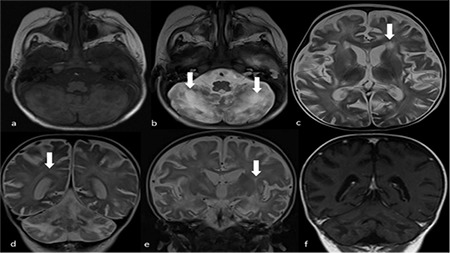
Griscelli syndrome: cerebral involvement. (a) Axial T1-weighted magnetic resonans (MR) image shows bilateral low-signal-intensity areas in white matter of cerebellum. (b) Axial fluid attenuation inversion recovery MR image demonstrates high-signal-intensity in this area. (c) Axial T2-weighted MR image at lateral ventricle level. (d, e) Axial and coronal T2-weighed images showing cerebral atrophy and diffuse high-signal-intensity in cerebral white matter. (f) Contrast-enhanced coronal T1-weighted MR image demonstrates no contrast uptake.
